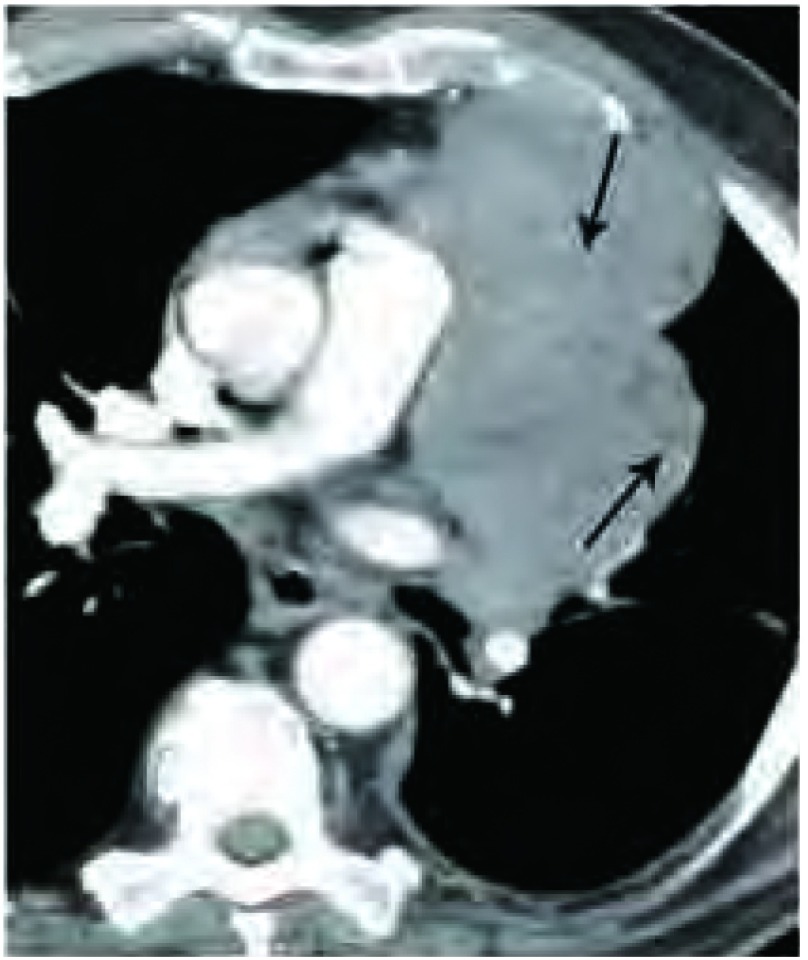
密度。胸部增强CT显示，肺动脉干水平的不均质胸腺瘤。箭头所示为肿瘤中多个低密度区中的两个。 Attenuation. Contrast-enhanced chest computed tomography (CT) (soft tissue window) of a heterogeneous thymoma at the level of the pulmonary trunk. Arrows point to two of the many small low attenuation regions within the mass.
